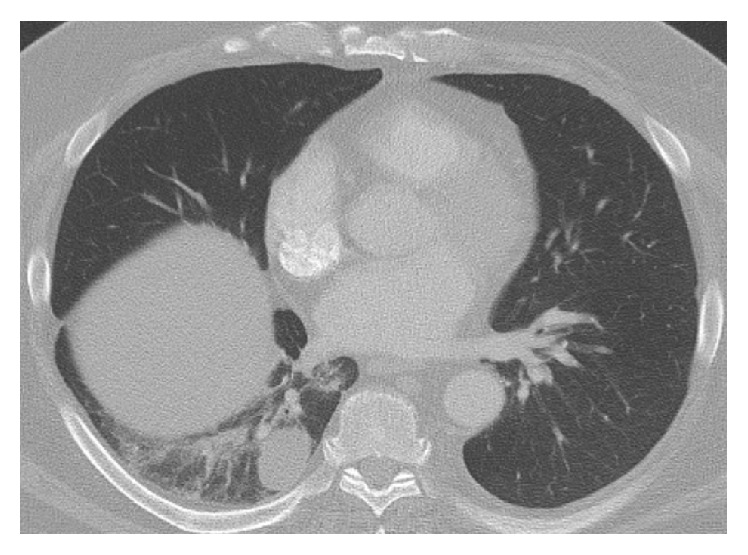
Axial image of CT of chest performed on April 15, 2015; pulmonary windows demonstrate an enlarging 3.6 cm nodular opacity in the right lower lobe with minimal subsegmental atelectatic changes and ground-glass opacity.
